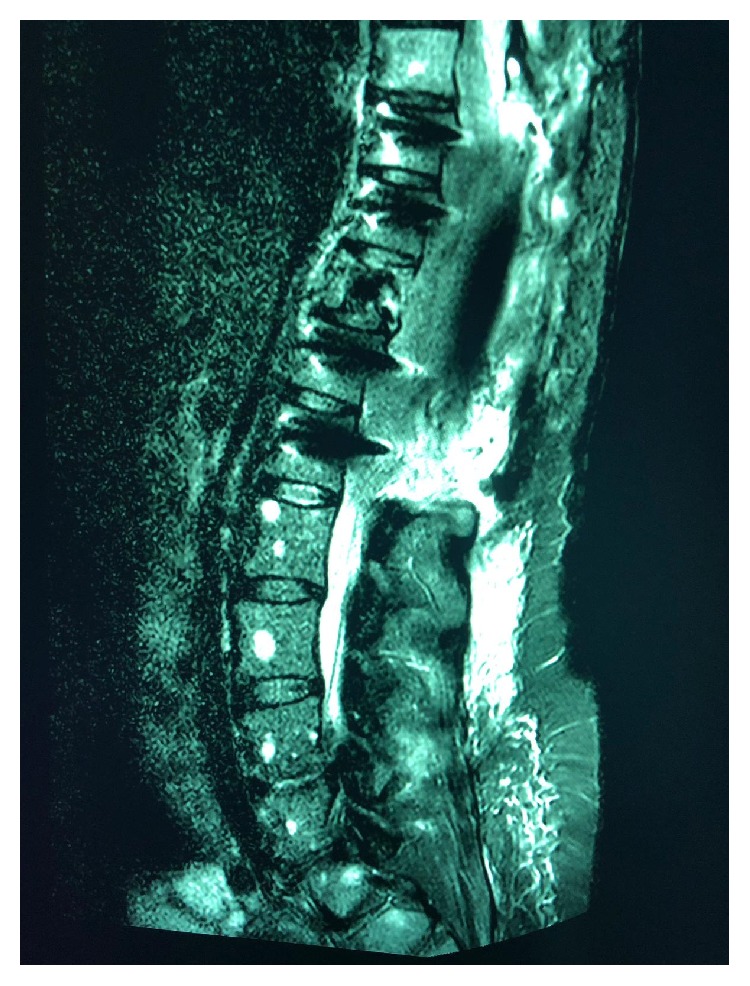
Post-spinal decompression and spinal fixation MRI.
